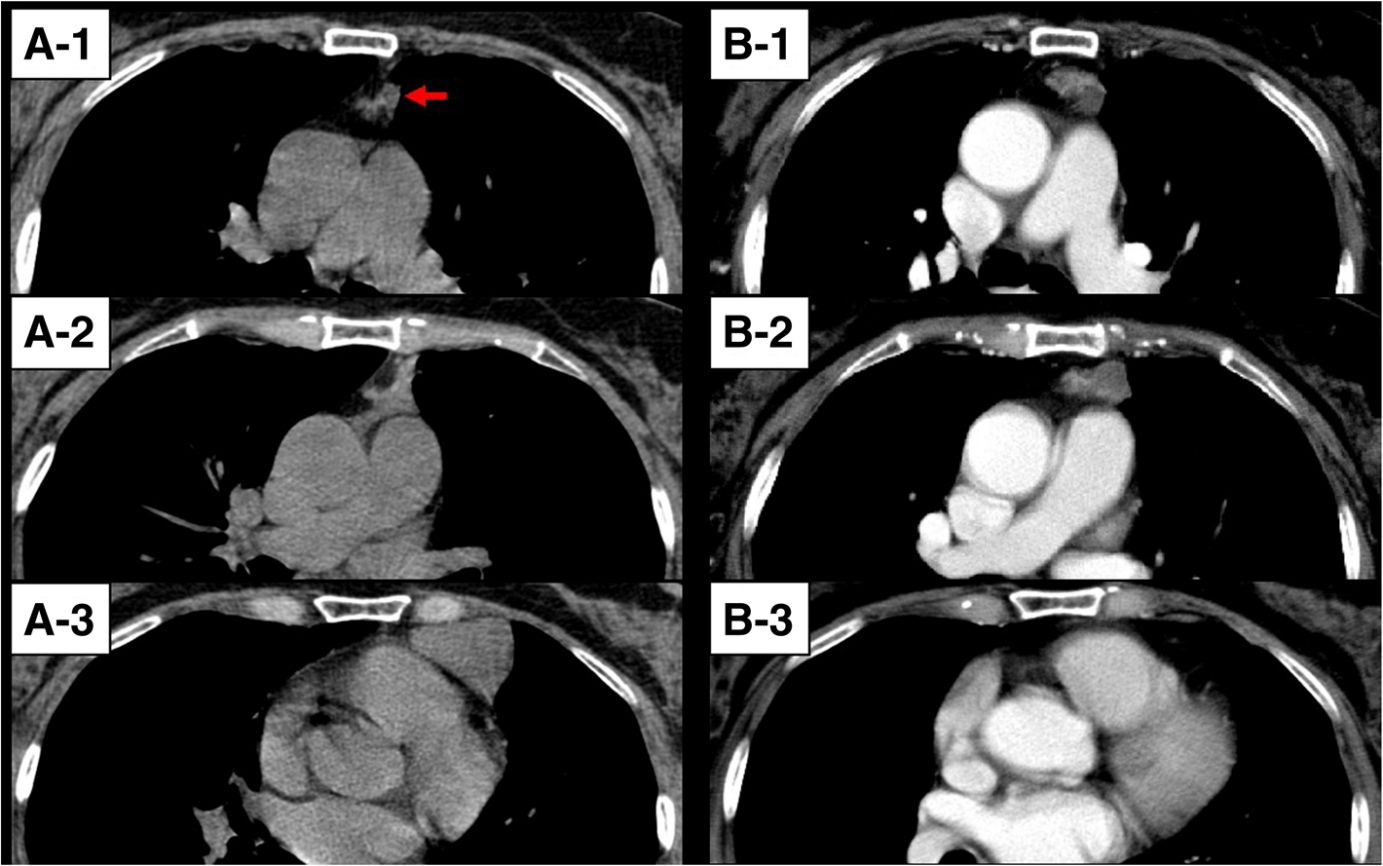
Computed tomography findings. a Retrospectively, chest computed tomography image which was taken before the previous operation shows not only cyst which was resected but also a small nodule (*arrow*) which was not resected at the anterior mediastinum. b Chest computed tomography imaging which was taken before the present operation shows heterogeneously enhancing mass at the anterior mediastinum. The mass seems to grow from the previously existed small nodule
